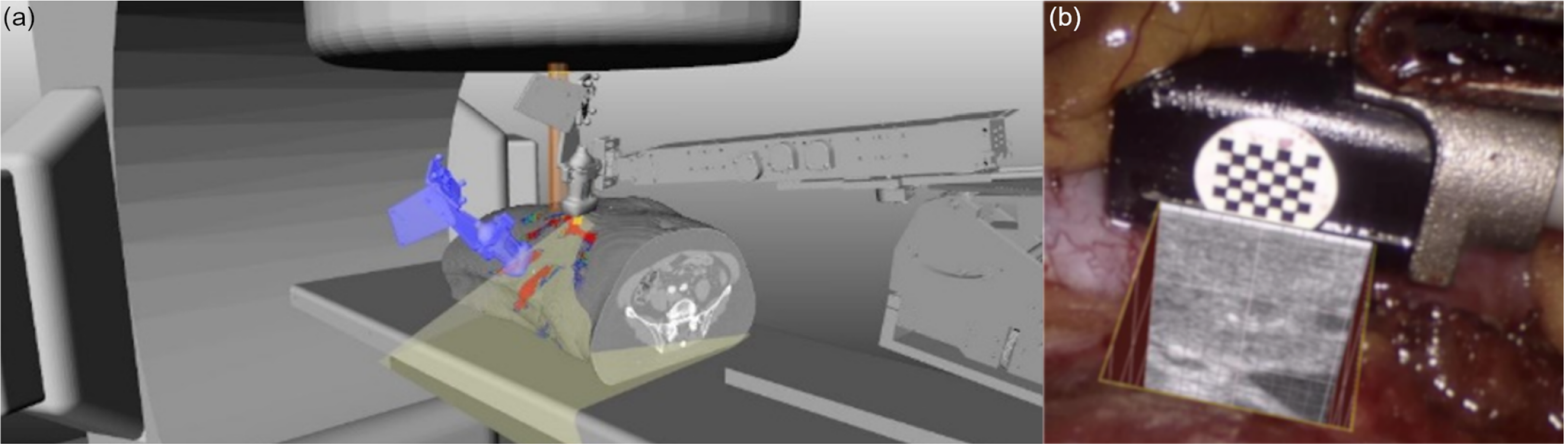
Examples of VR and AR in robotic ultrasound. a Virtual radiotherapy scenario showing a linear accelerator and the robotic ultrasound acquiring data from a patient (copyright [2016] John Wiley & Sons, Inc. Used with permission from [78] and John Wiley & Sons, Inc.). b 2D ultrasound image superimposed on a laparoscopic video image (reprinted from [79], copyright [2014] with permission from Elsevier)
